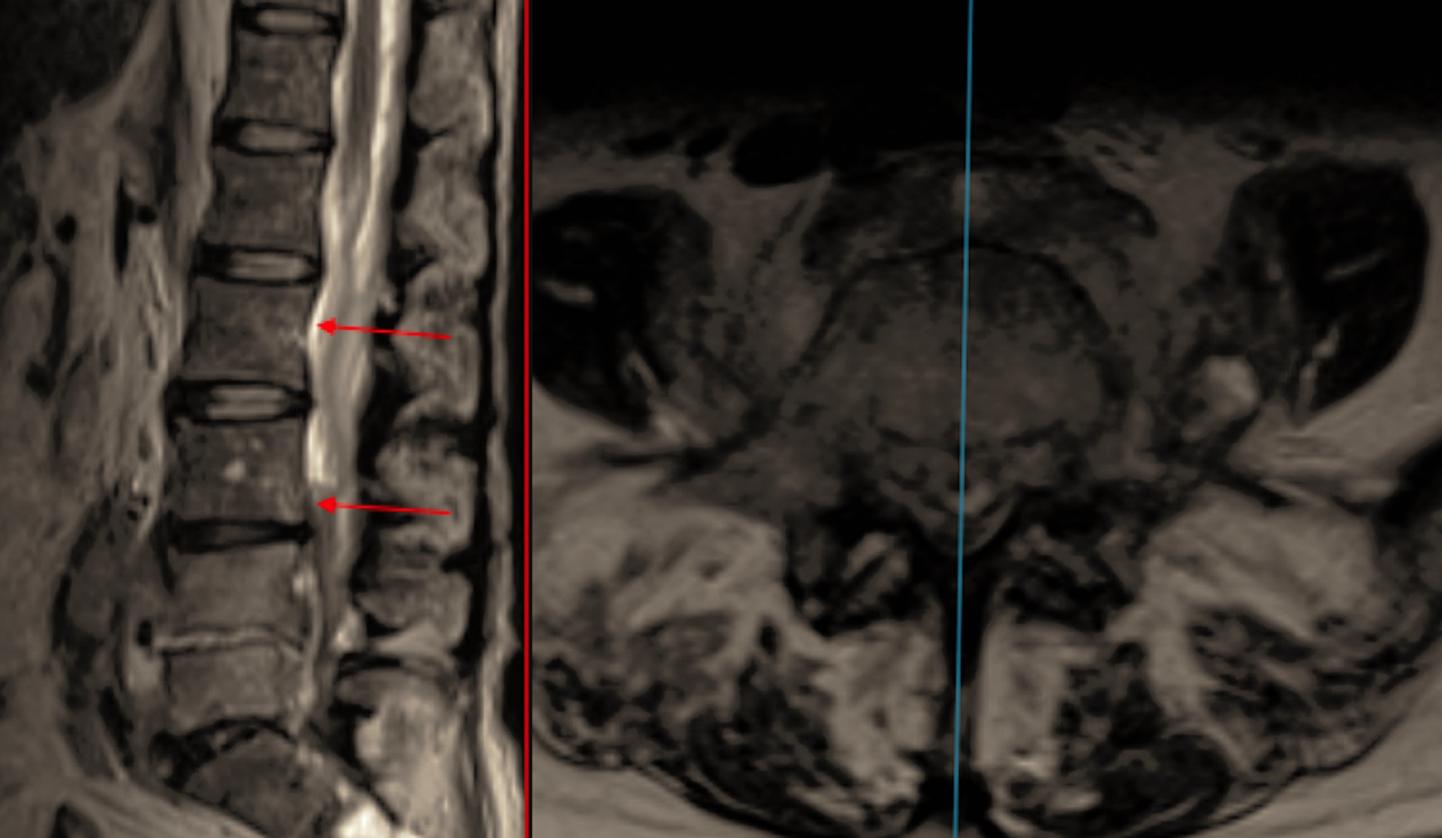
T2 TSE Sagittal and Axial MRI showing worsening of the epidural collection with proximal extension (red arrows). TSE - Turbo Spin Echo; MRI - Magnetic Resonance Imaging
